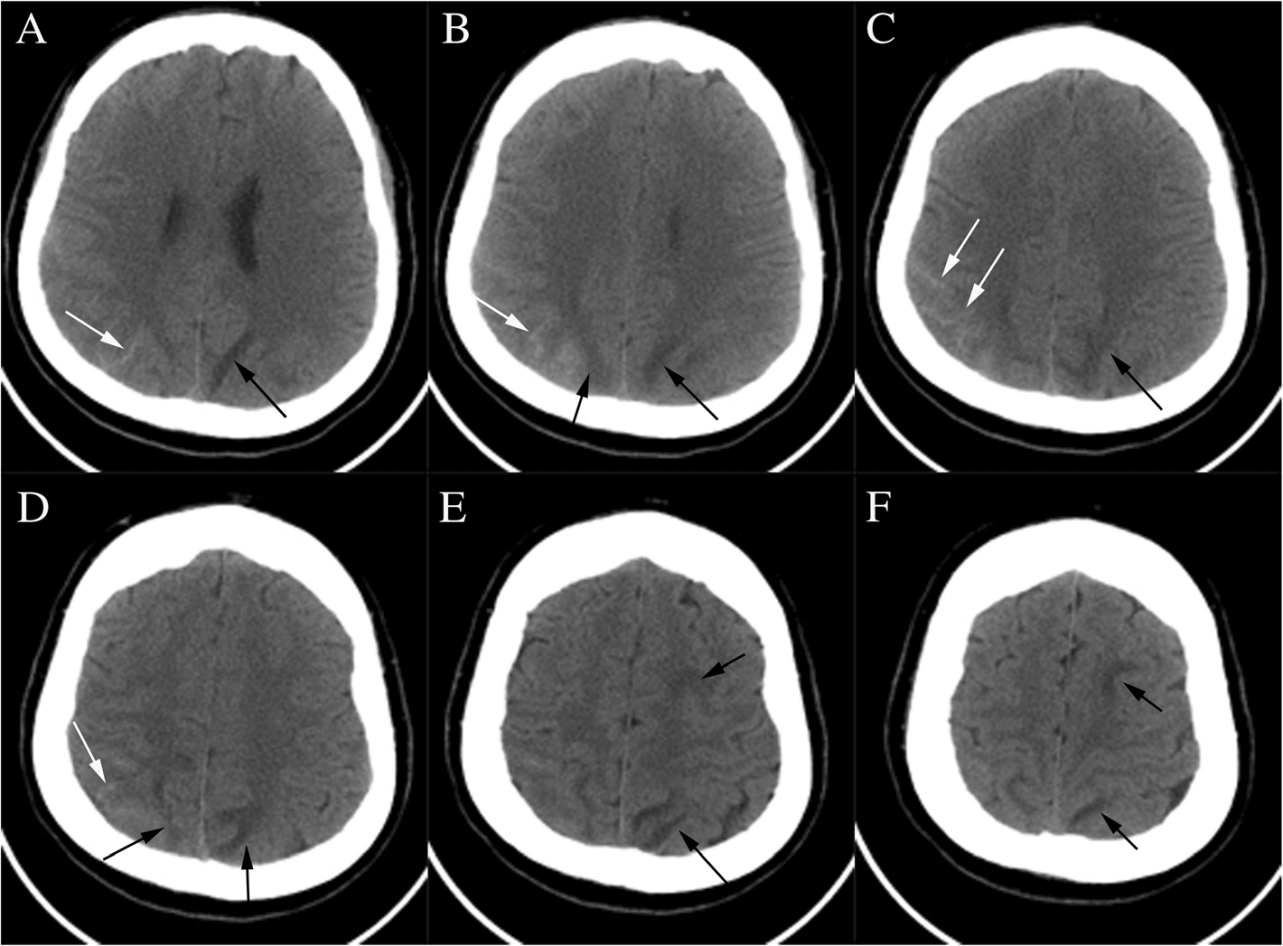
CT scan (a-f) shows lesions with hyperdensity in the parietal-occipital sulci (white arrows) and hypodensity in the left frontal and parietal lobes (black arrows)
